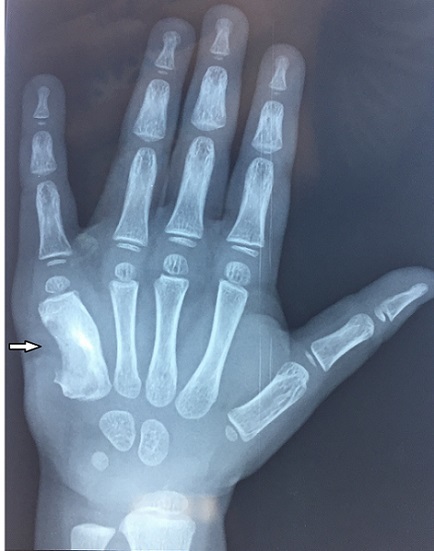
X-ray of right hand showed an expansile, cystic and lytic lesion with cortical erosion involving the little finger metacarpal
